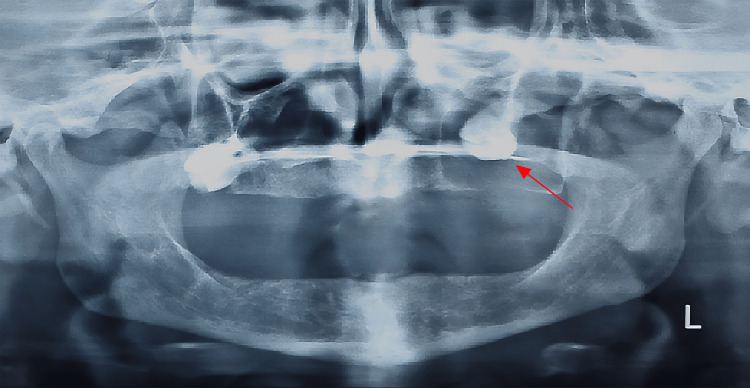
Panoramic radiograph examination. An impacted upper left third molar into the left maxillary sinus (red arrow) with bone resorption and complete pneumatization of the sinus inferiorly till the alveolar crest level was noticed.
